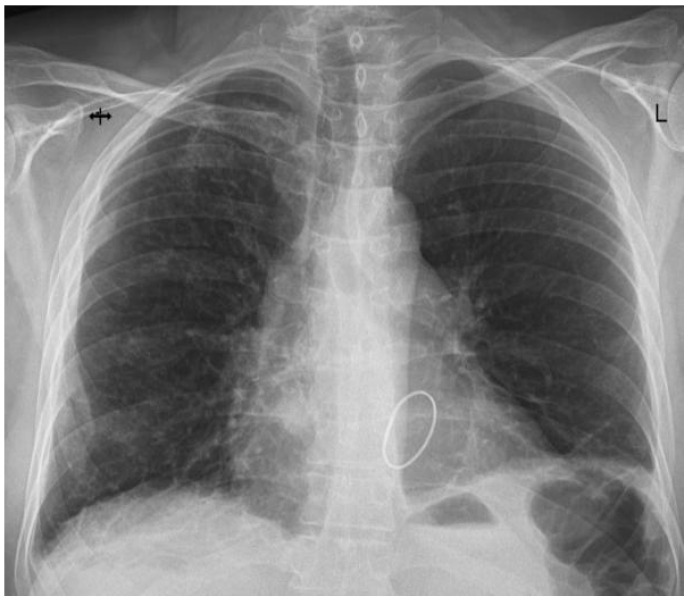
Thoracic X-ray at one month follow-up.
